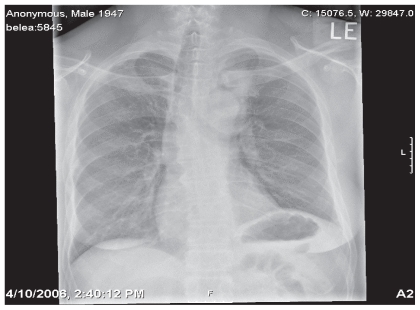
Chest X-ray showing superior mediastinal mass.
